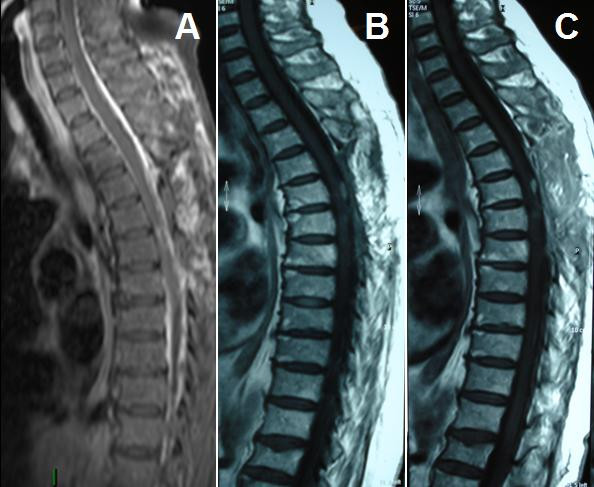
Postoperative MRI: Sagittal T1 weighted image with gadolinium 10 days (a), 3 months (b), and 10 months (c) after surgery.
